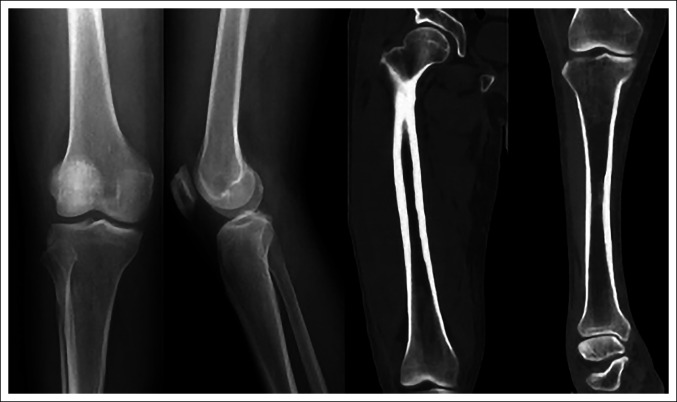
Radiograph and CT showing no obvious abnormalities in lower extremity.
